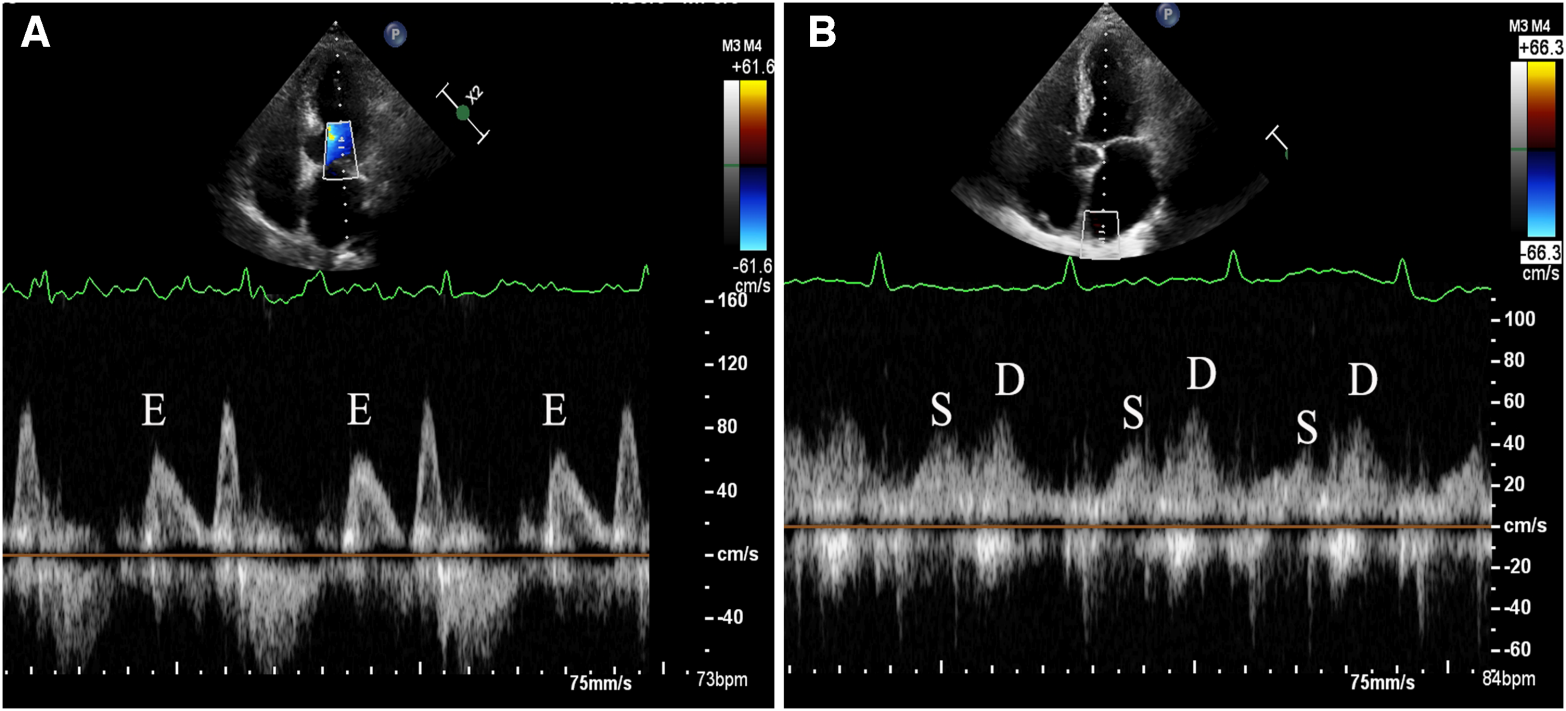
Demonstration of measurement method for left atrial acceleration factor (α) by transthoracic echocardiography. (A) The apical 4-chamber view was used to measure the peak velocities of the diastolic early (E) trans-mitral Doppler flow, placing the sample volume at the tip of the mitral leaflets. (B) The maximum inflow velocities of the LA were defined using the recorded velocity of the right upper pulmonary vein inflow during systole (S) and diastole (D).
